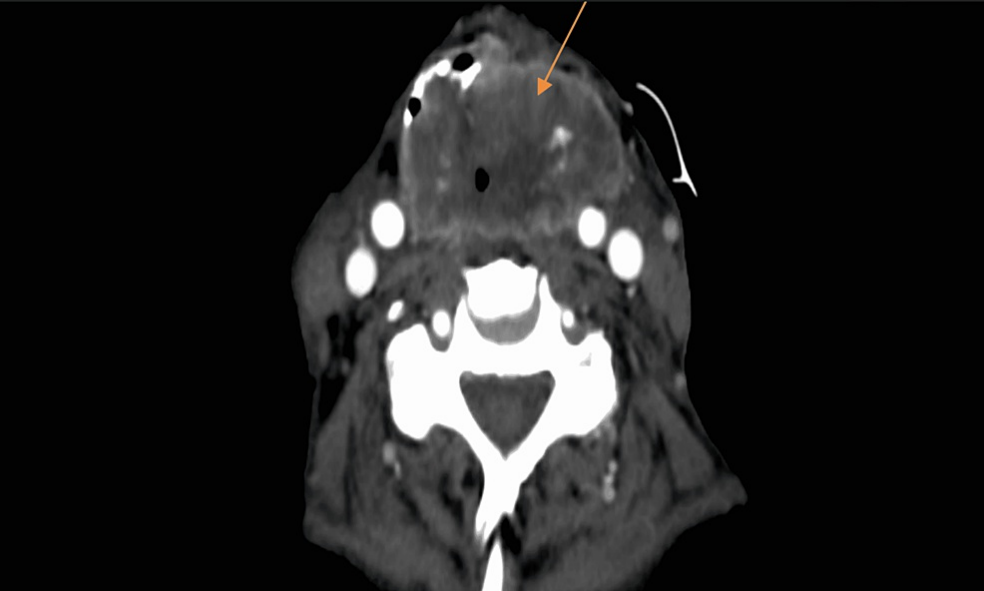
CT soft tissue neck with contrast with lobulated irregular enhancing mass in the expected location of pharynx and larynx
